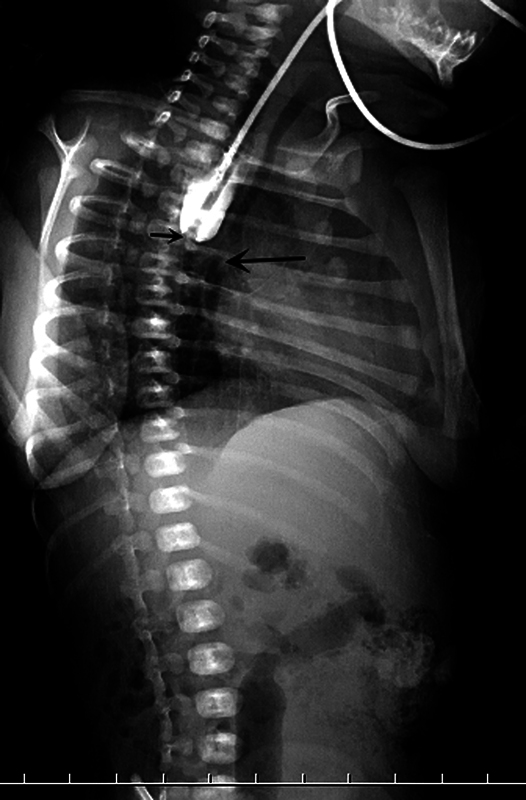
Chest radiograph on lateral view showed the orogastric tube inserted at the proximal esophageal pouch (
